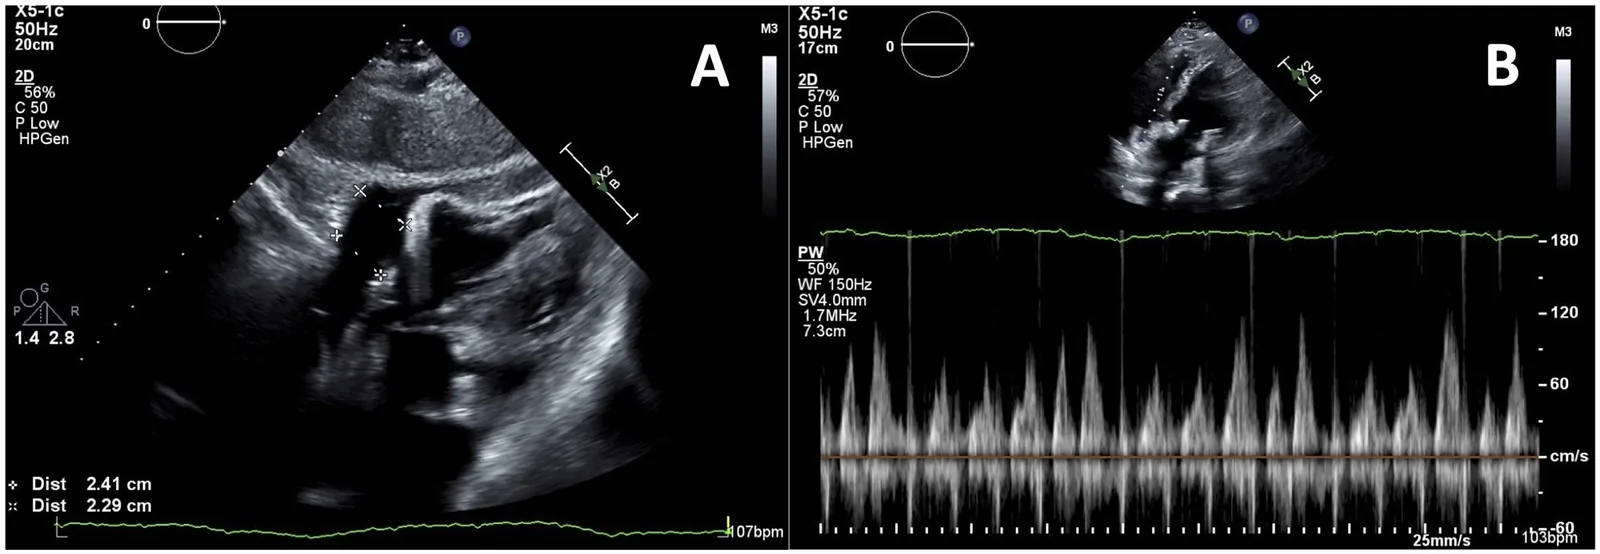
TTE demonstrating a focal pericardial effusion adjacent to the right ventricle’s free wall (A). Pulsed-wave Doppler of the mitral valve inflow demonstrating marked respiratory variation (B).
